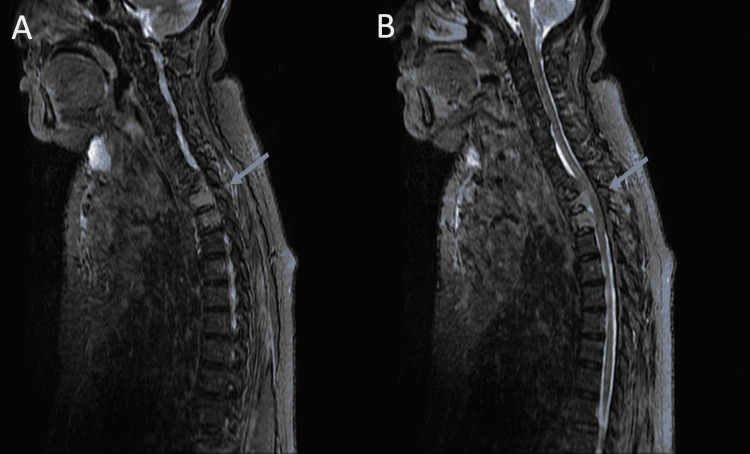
(A&B) Sagittal images of T2-weighted MRI of the thoracic spine with contrast. Figure A with radial blue arrow showing infiltrating and destructive epidural lesions at T2 and T3 vertebral bodies in keeping with metastatic disease. Figure B with radial blue arrow pointing towards thoracic cord segment compression at T2 and T3 levels caused by likely metastatic lesions.
